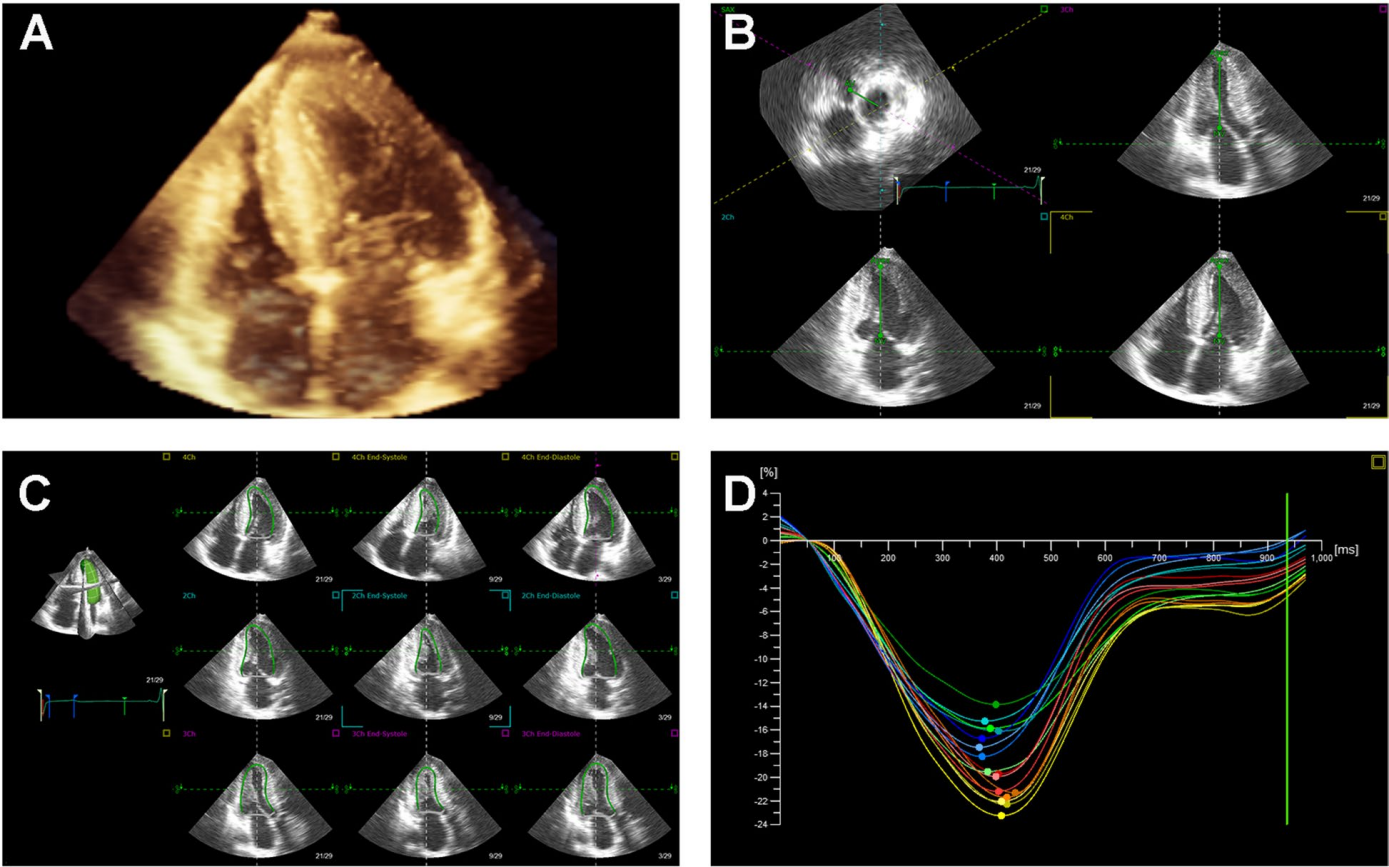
3D STE offline analysis. A An apical four-chamber 3D full volume image. B The points of the cardiac apex and mitral valve are manually adjusted for software automatic identification. C The endocardial border is traced and tracked in the apical triplane views. D An example of GLS obtained from 3D STE analysis
